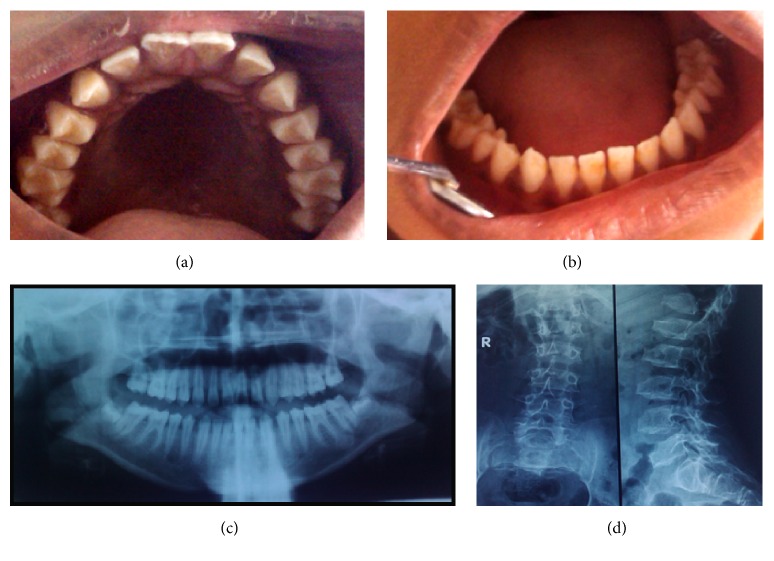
(a), (b) Spade-shaped incisors, pointed cusps, and spacing between teeth in case 1. (c) Orthopantomograph showing thin enamel with normal radio density. (d) Spinal radiographs revealing kyphoscoliosis and tongue-like projections from anterior surface of vertebral bodies in case 1.
